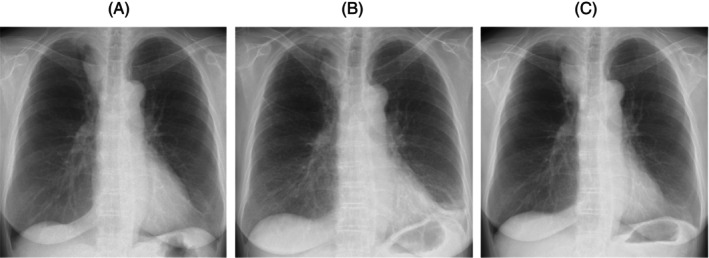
(A) Chest radiography before the introduction of sotorasib showing no ground glass opacities in both lung fields. (B) Chest radiography on day 80 after the start of sotorasib reveals ground glass opacities in both lung fields with decreased lung volume. (C) After prednisolone treatment, ground glass opacities in both lung fields disappear and lung volume improves, but the mass shadow in the right upper lung field increased.
